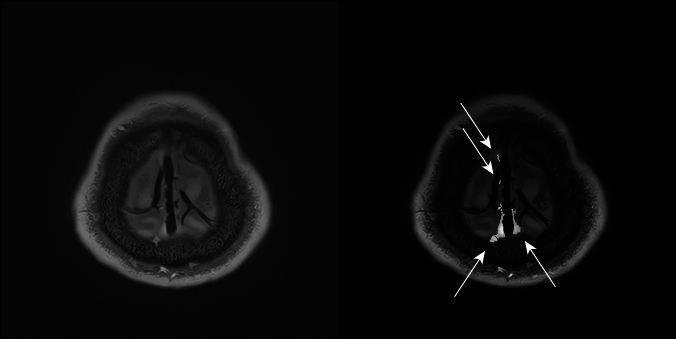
High-signal areas adjacent to the superior sagittal sinus drawn as regions of interest (*arrows*) on motion-sensitized driven equilibrium (MSDE) T1-weighted transverse images
